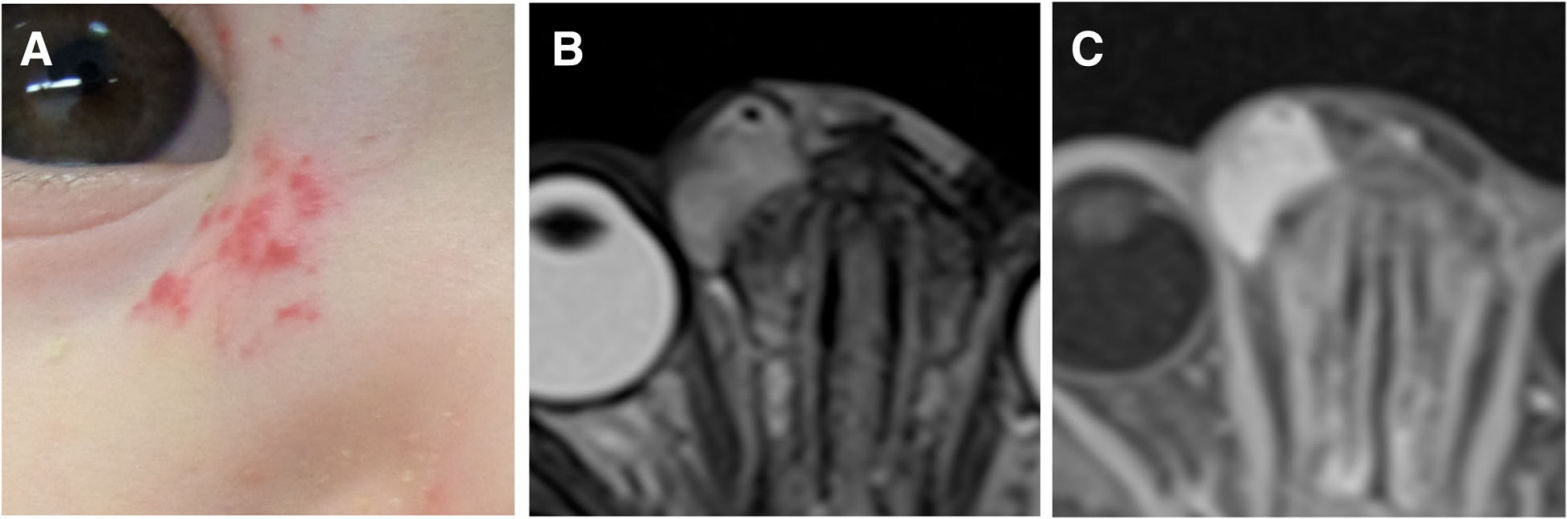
Infantile hemangioma in an 8-month-old boy. The tumor is superficially visible through disseminated red papules (a). On MRI, the tumor showed a typical homogeneous signal on T2w (b) and a corresponding homogeneous enhancement after Gadolinium administration (c)
